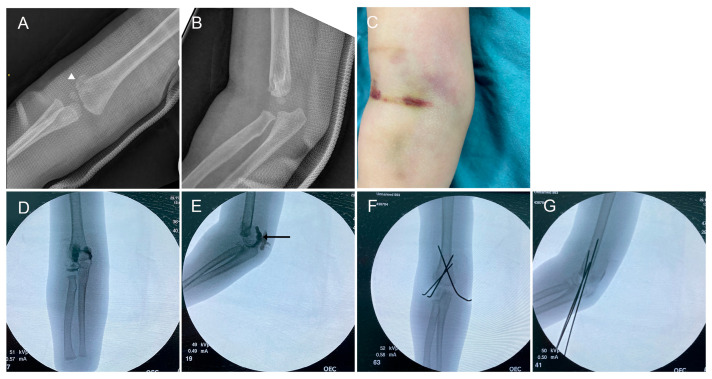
Transphyseal distal humerus fracture (TFDH) misdiagnosed as a lateral condyle fracture. A 17-month-old girl (Case 17) was initially misdiagnosed with a lateral condyle fracture. (A,B): Initial anteroposterior and lateral radiographs. White arrowhead denotes the metaphyseal (Thurston–Holland) fragment. (C): Soft tissue swelling of both the medial and lateral aspects of the elbow is present. (D,E): Arthrograms show posterior displacement of the distal humeral epiphysis with intact articular cartilage (black arrows). (F,G): Closed reduction and percutaneous pin fixation performed under arthrography guidance.
